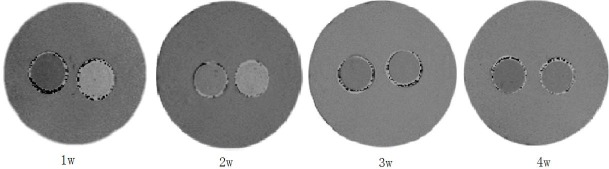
MRI results of BMSCs_-FTH1_ and BMSCs on 7T MRI device. (A) As revealed by the T2-mapping images of BMSCs_-FTH1_ and control BMSCs acquired by 7T MRI, the signal intensity of BMSCs_-FTH1_ (left) was darker than that of BMSCs (right).
